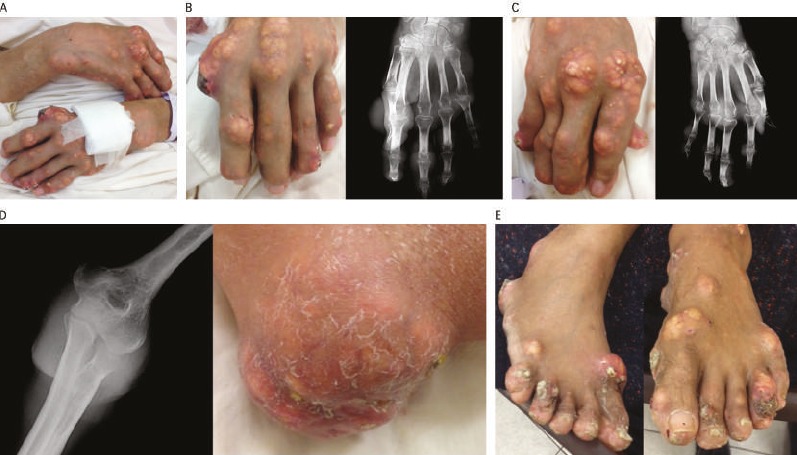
Physical Examination Findings and Description Non-tender tophi from 1 to 6 cm were obvious at inspection. A–C. Wrists, metacarpophalangeal, proximal and distal interphaiangeal articulations. D. Elbows. E. Ankles and first metatarsophalangeal joint. Radiographs of upper and lower extremities revealed bone erosions with the characteristic ‘overhanging’ edges.
